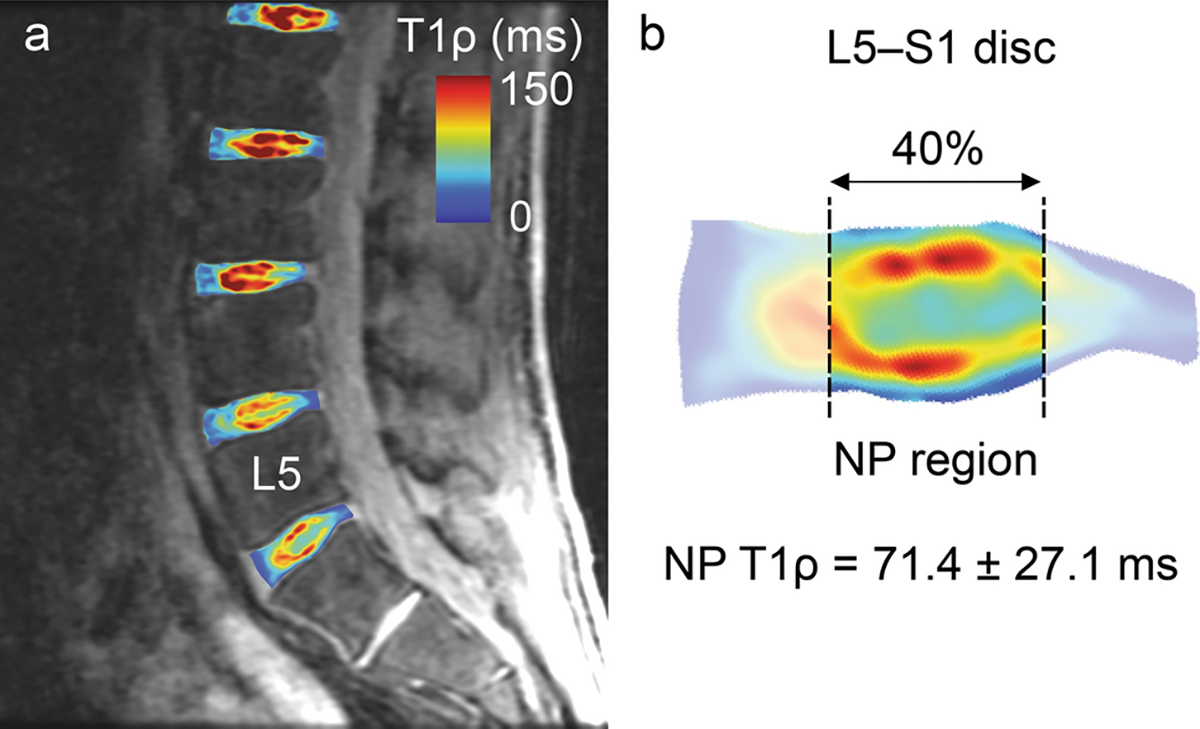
a Mid-sagittal T1ρ MRI image showing lumbar disc segmentations and relaxation time maps. b The mean ± SD T1ρ relaxation time was computed in the nucleus pulposus (NP) region of each disc
